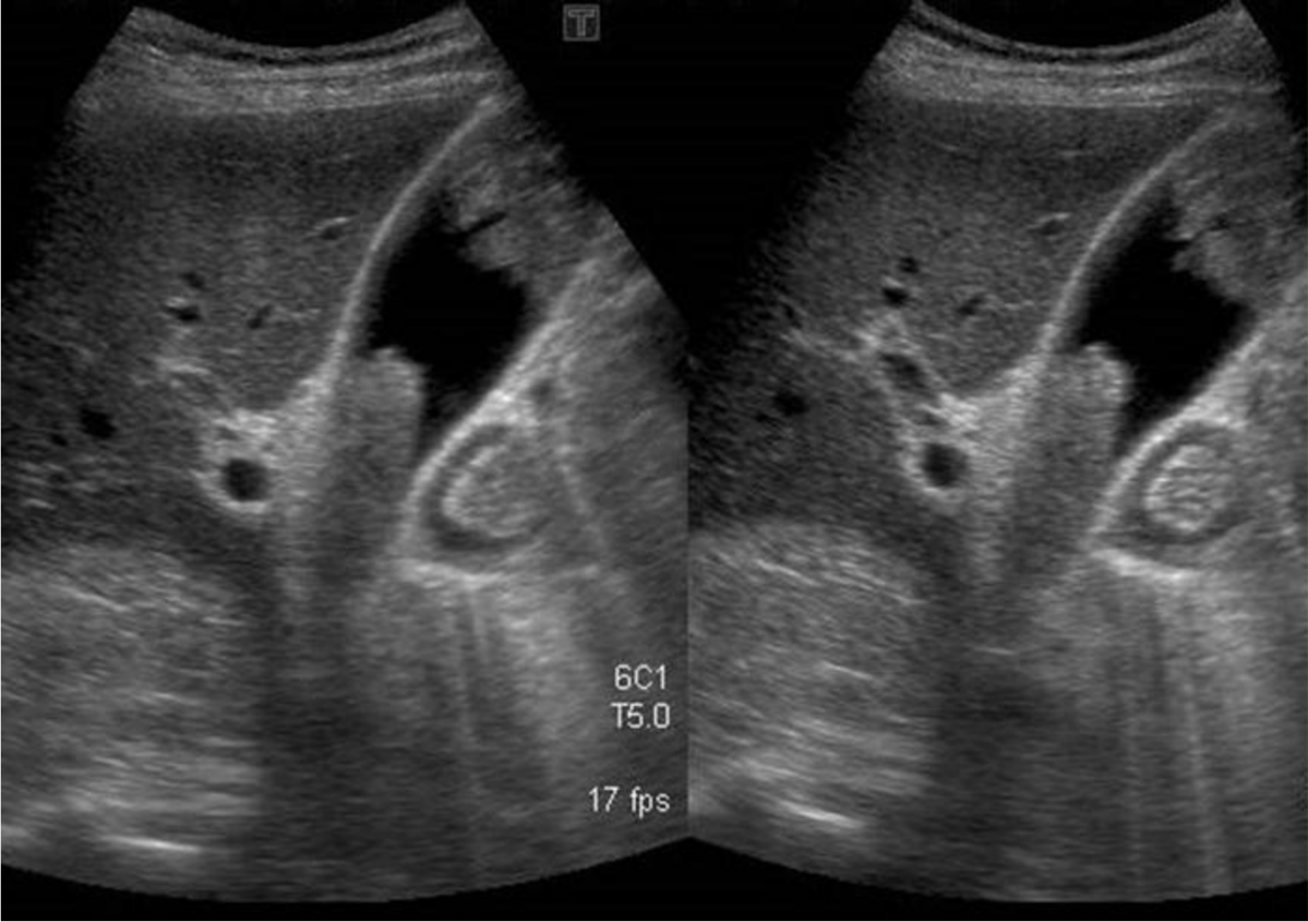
Hepatobiliary ultrasound. Hyperechoic masses involving the gallbladder fundus, body and neck; the parietal lesions with minimal to absent acoustic shadowing.
